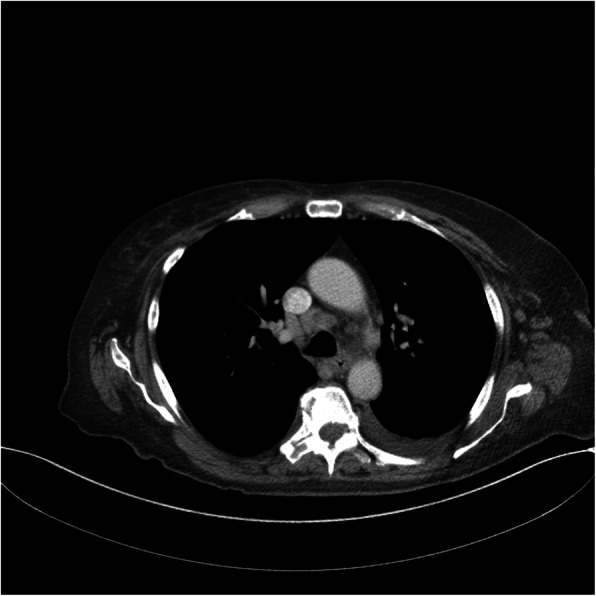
Computed tomography scan of chest. Multiple mediastinal lymph nodes are present with the largest one measuring 1.75 × 1.0 cm
